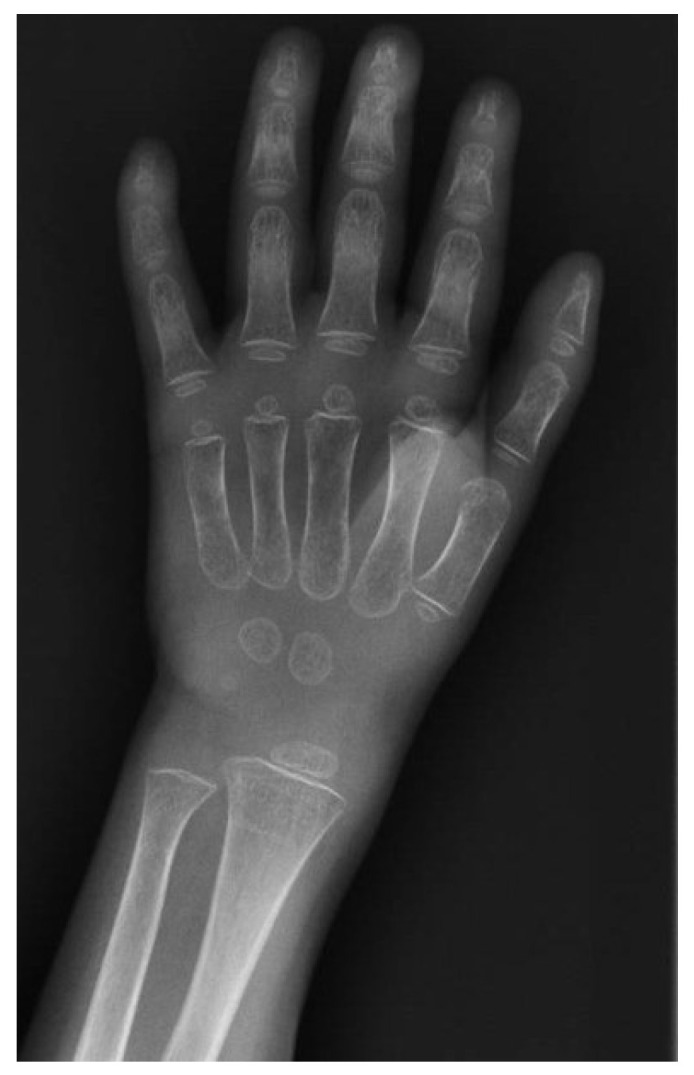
Wrist X-ray showing severe bone demineralization.
